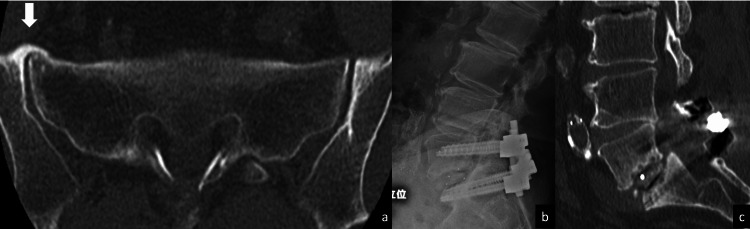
Case 3: A case with anterior bony ankylosis of the right sacroiliac joint. Bone fusion was not achieved even one year after surgery: (a) Preoperative axial CT slice showing bony ankylosis of the right sacroiliac joint. The white arrow indicates the bony bridge. (b) Postoperative X-ray during extension showing the cleft around the cages. (c) Postoperative sagittal CT showing the cleft above the endplates and vertebral endplate cysts.
